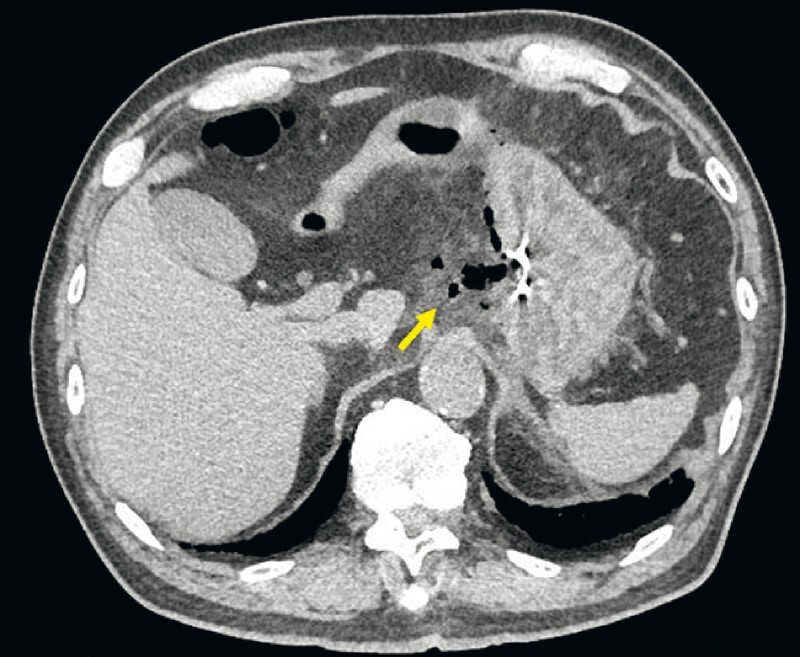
Computed tomography, taken on postoperative day 1, shows free air and inflammation (arrow) in the adjacent area of the stomach.
